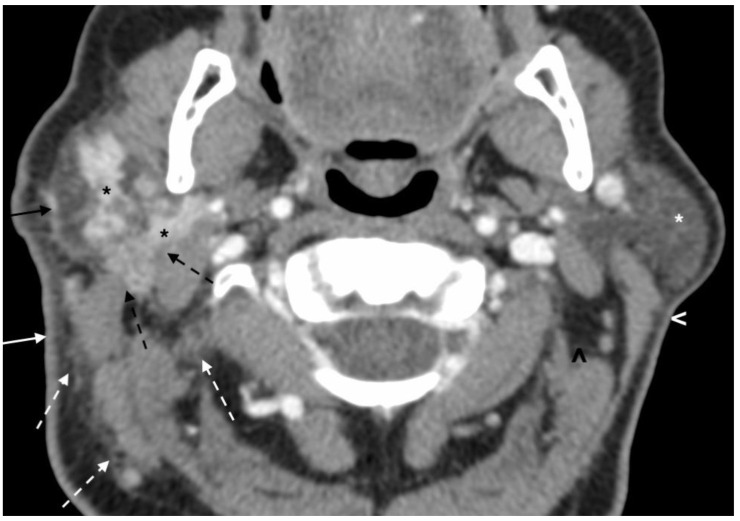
Initial post-contrast CT scan of the neck demonstrates a nodular and infiltrative lesion (black asterisks) centered in the right parotid gland, and significant surrounding fat stranding and fat plane effacement (dashed white arrows) involving the adjacent neck spaces and structures. Both the right sternocleidomastoid and posterior belly digastric muscles have ill-defined margins with the lesion (dashed black arrows). Additionally, there is thickening of the fascial plane (solid black arrow) and overlying skin (solid white arrow). On the contralateral side, there is maintenance of the fat planes (black arrowhead), normal skin (white arrowhead) and fascial plane thickness, and normal CT scan appearance of the parotid gland (white asterisk).
